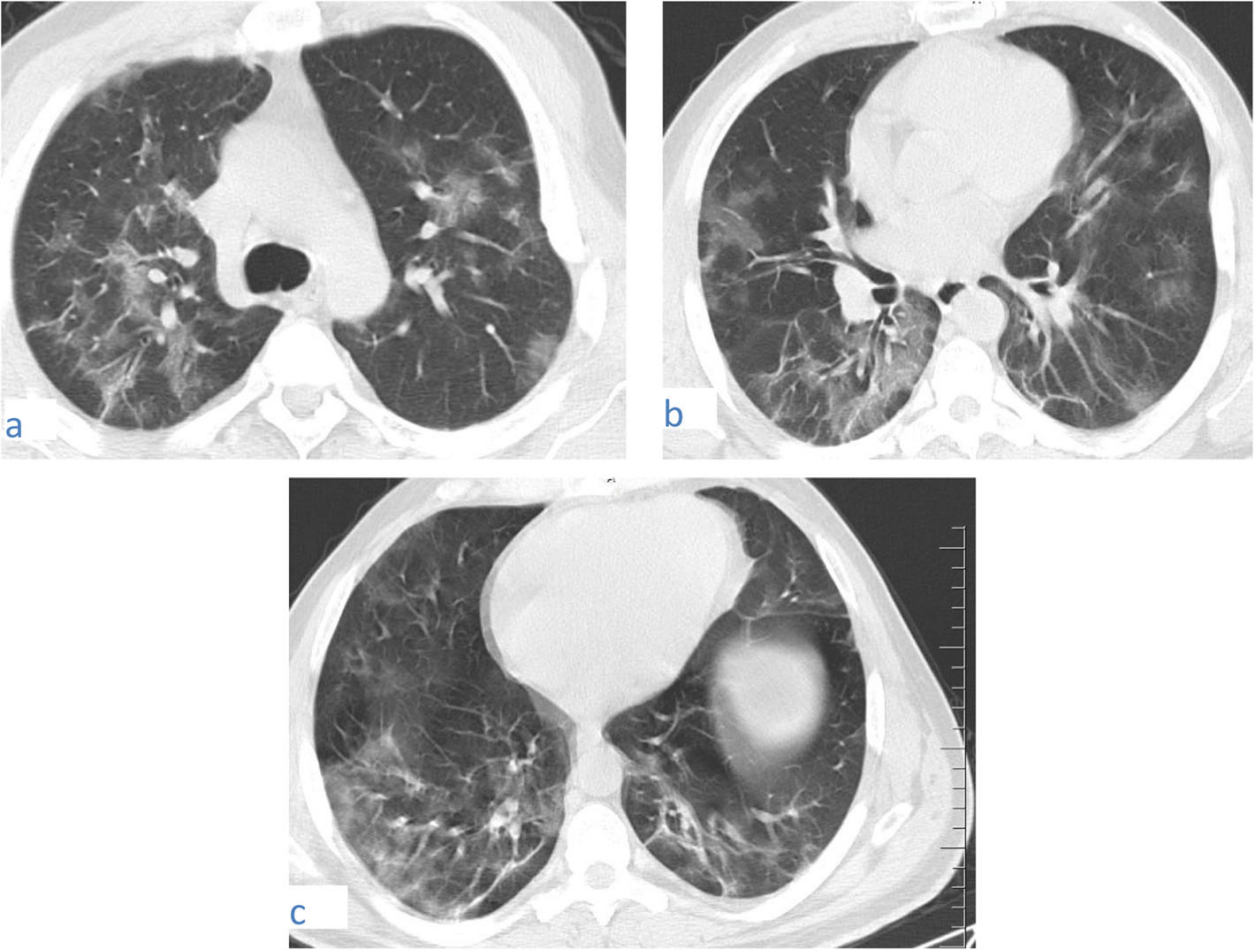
A 38-year-old male patient with COVID-19. CT chest axial images (a), (b), and (c) show subpleural and patchy ground glass opacities with vascular thickening, and show bilateral basal subpleural areas of ground glass opacities, fibrous stripes, architectural distortion, and perilobular thickening
